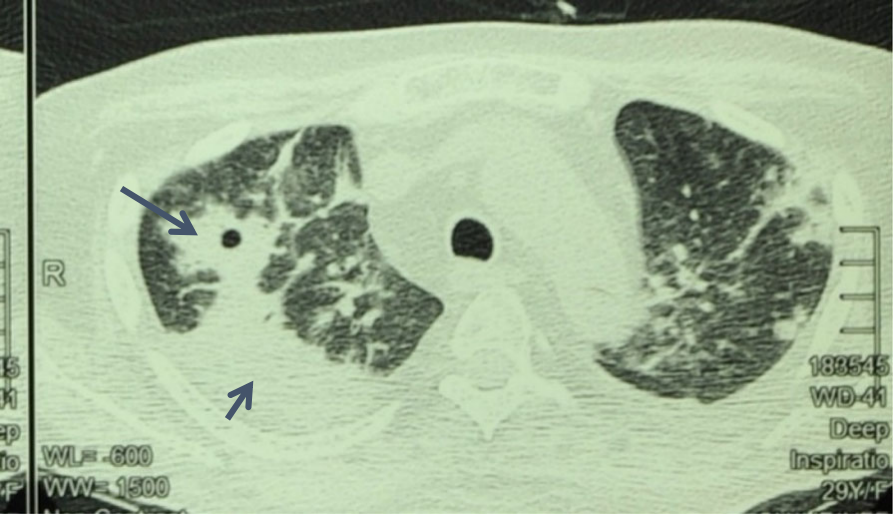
Contrast CT chest showing consolidation nodules and cavitation of upper lobe of the right lung (long arrow: cavity, short arrow - consolidation)
